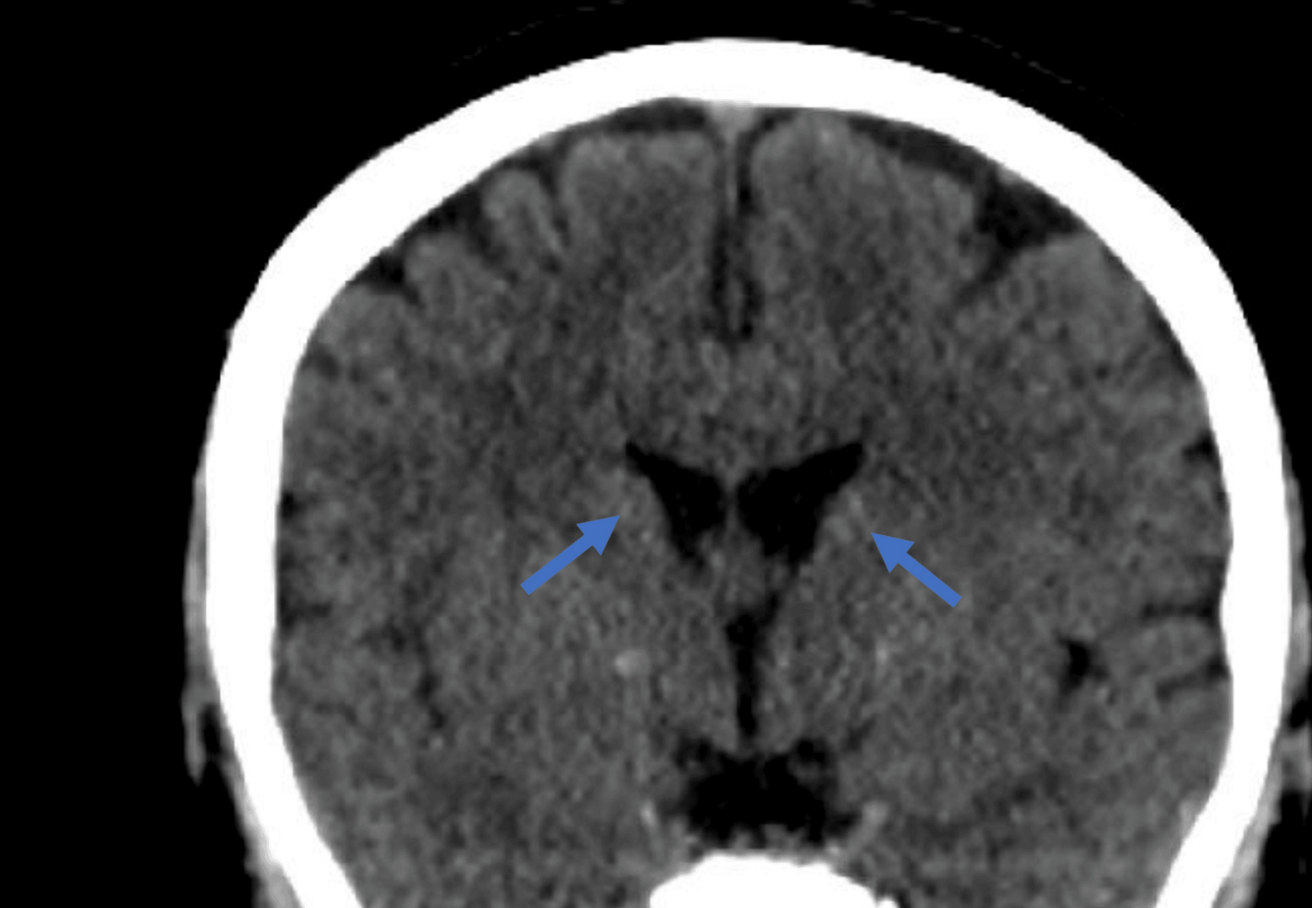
CT scan of the brain without contrast Bilateral basal ganglia (globus pallidus and head of the caudate nucleus) exhibit spotty calcifications (blue arrows).
